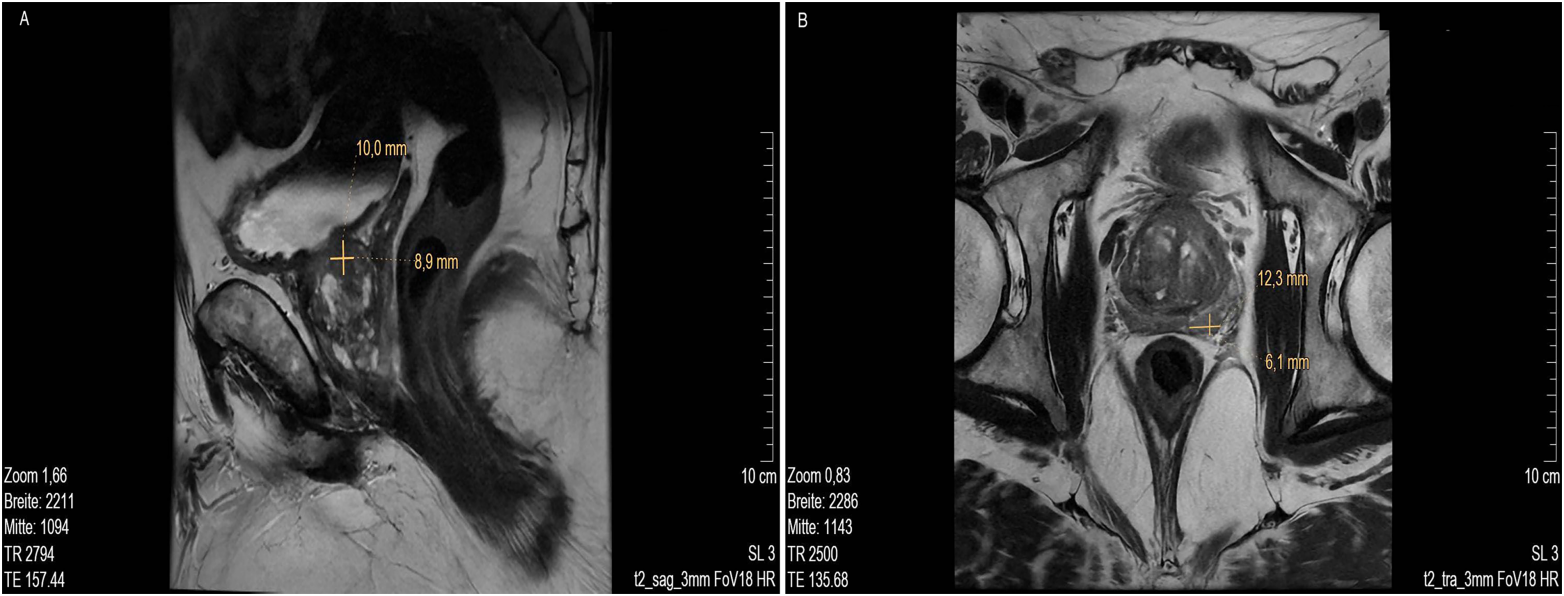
MRI scan lower pelvis (A) MRI sag T2 3 mm FOV 18, showing lesion 1 at about 9- to 10-o’clock position (marked with cross). (B) MRI low signal in T2 tra 3 mm FOV 18 with lesion 2 at approx. 5- to 6-o’clock position in the peripheral zone, close to the capsule, about 11 mm in length (marked with cross). MR images courtesy of Dr. med. Stefan Oehm from die Radiologen, Weiterstadt, Germany. MRI, Magnetic Resonance Imaging; sag, sagittal; FOV, field of view; tra, transverse
